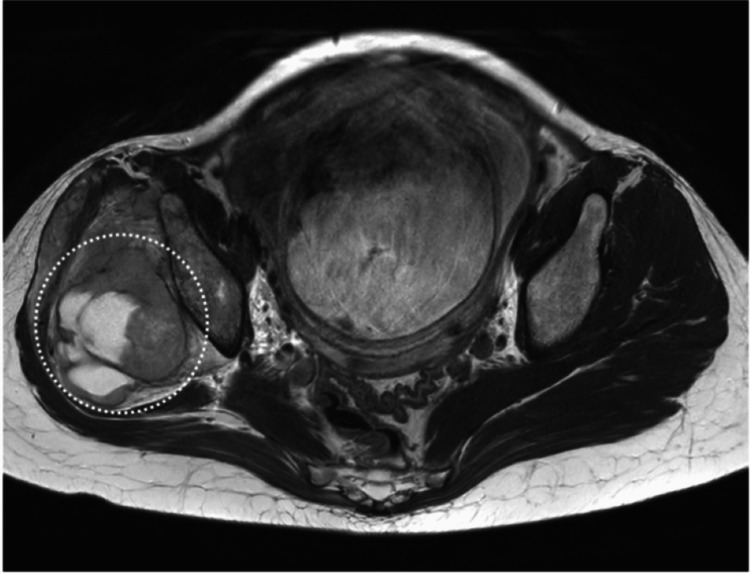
T2-weighted MRI obtained at admission MRI scan showing a large synovial sarcoma (white dotted circle) in the right gluteal region with involvement of adjacent soft tissues.
